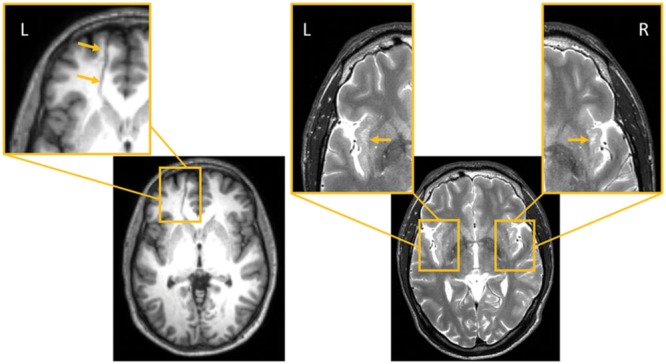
(Left): Subject 1. (1) Axial FLAIR MRI image shows a transmedullary vein close to the head of the left caudate nucleus crossing the medial frontal lobe substance and reaching the superior longitudinal sinus. This region has been expanded to depict the venous angioma (orange arrows; left panel, inset). (Right): Subject 2. (2) Axial T_2_-weighted axial MRI imaging shows enlarged perivascular spaces in both insular cortices; the largest one are in the left anterior insula (orange arrows, insets). L indicates left and R right sides of the brain.
